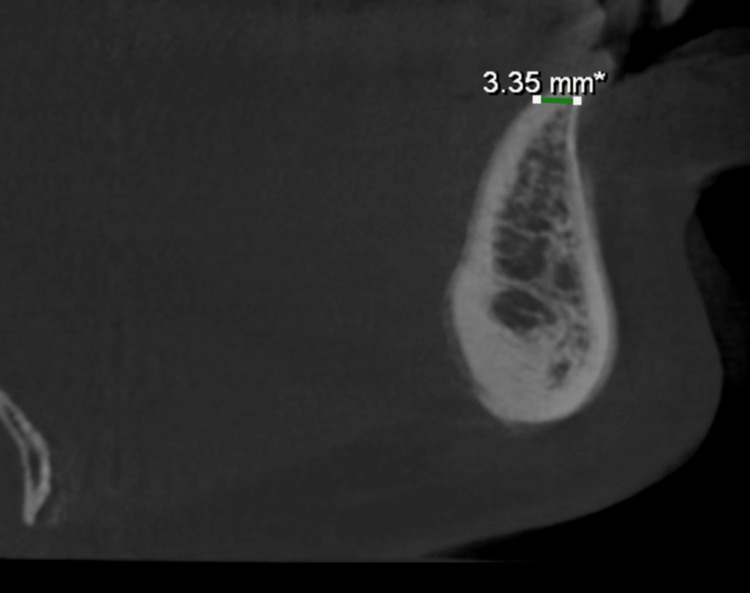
CBCT (sagittal view) showing a preoperative mandibular ridge width of 3.35 mm. CBCT, cone-beam computed tomography
